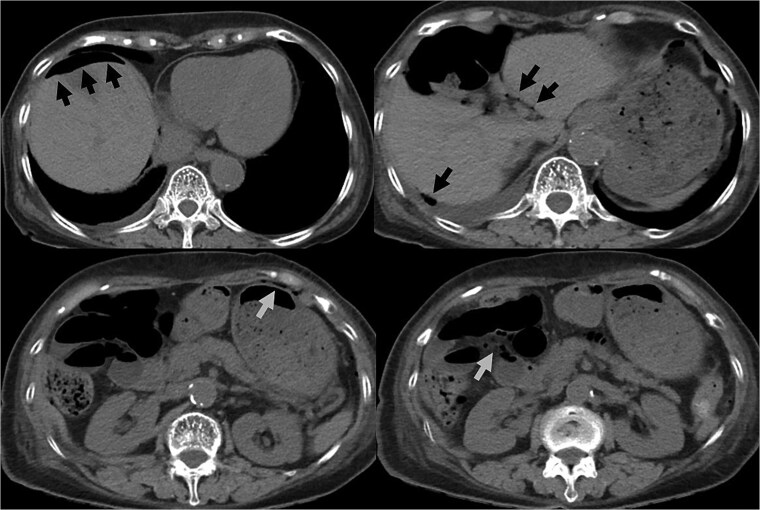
CT indicated intra-abdominal free air (arrows). These findings were later confirmed as being due to bladder rupture.
